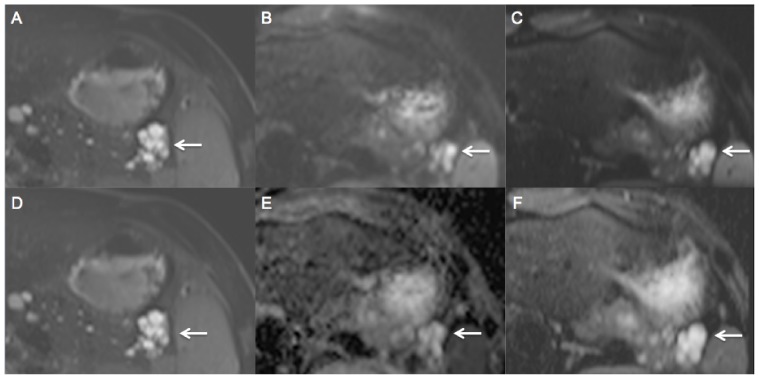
A 27-year old patient with serous cystadenoma in the pancreatic tail (arrow). T2 HASTE (A, D), c-EPI DWI (B, E) and z-EPI DWI (C, F) of the pancreas were acquired. z-EPI DWI (C) and zoomed ADC map (F) demonstrate a better delineation of the pancreas and the serous cystadenoma compared to c-EPI DWI (B) and conventional ADC map (E).
